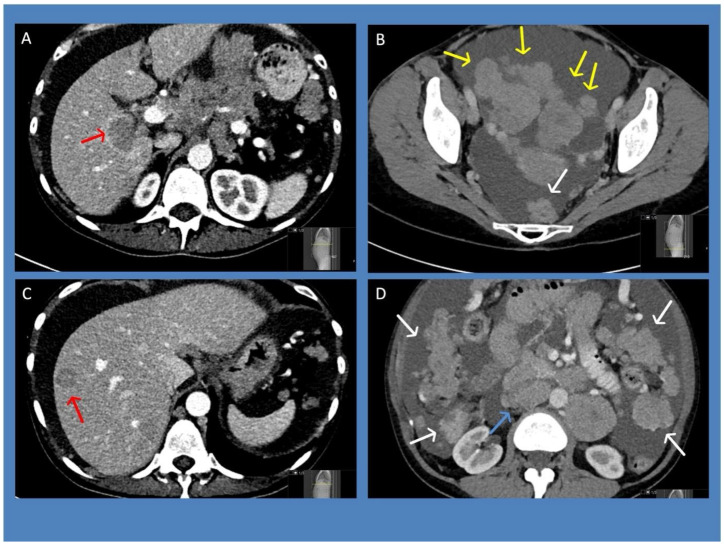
Stage determinant CT findings in a stage IVB EOC. (A,C) show liver metastases (red arrows). (B) shows bilateral primary malignant ovarian tumours (yellow arrows) and a peritoneal implant in the Douglas fossa (white arrow). Figure (D) shows bilateral peritoneal and omental implants (white arrows) and a retroperitoneal lymph node metastasis (blue arrow).
